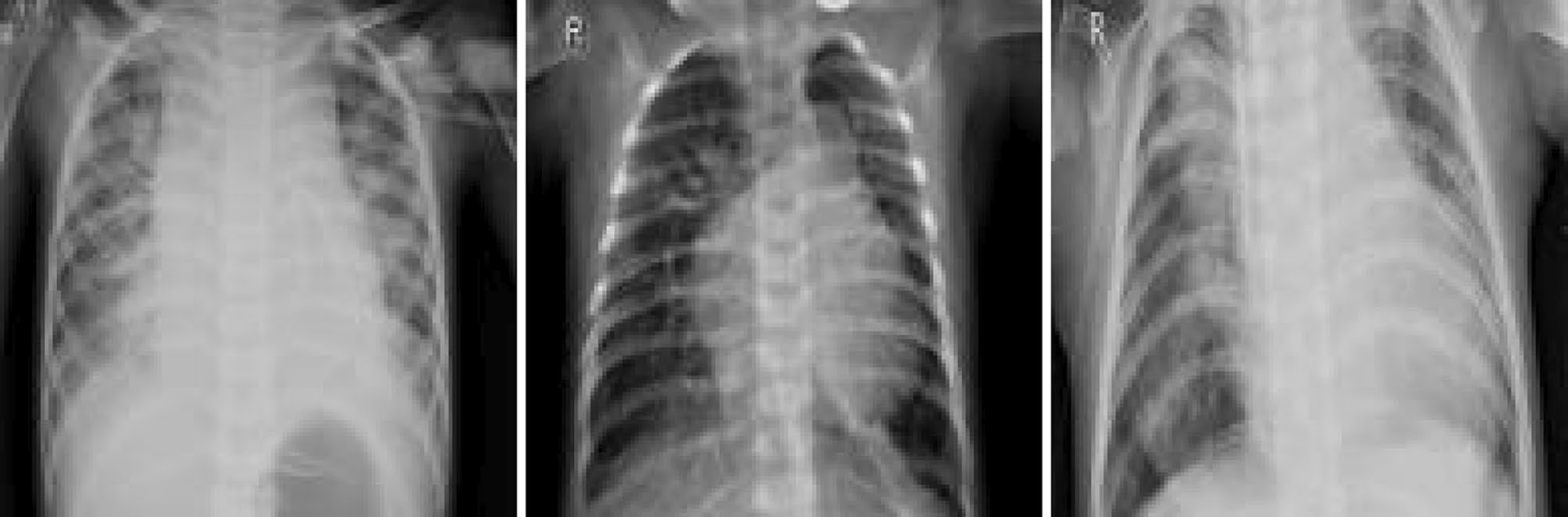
Some randomly sampled X-rays (with a size of 128 × 128) containing pneumonia.
